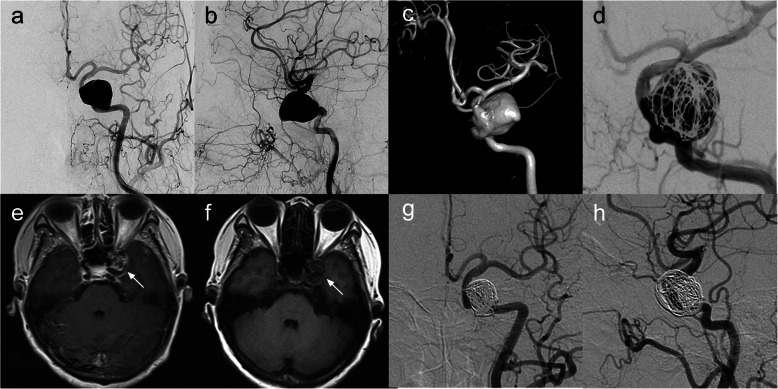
Images from a patient with a left large cavernous carotid artery aneurysm (patient 1). a–c Preoperative angiograms of the left internal carotid artery showing a large cavernous carotid artery aneurysm. d Immediately postoperative angiogram of the left internal artery showing reconstruction of the parent vessel and contrast stasis in the lumen of the aneurysm. MRI 4 months posttreatment (f) compared with the 2-day posttreatment MRI (e) showing a slight reduction of the aneurysm size (white arrow) and increased space around the brainstem. g, h Angiogram 66 months posttreatment showing the occluded aneurysm and reconstruction of the left internal carotid artery. MRI, magnetic resonance image
